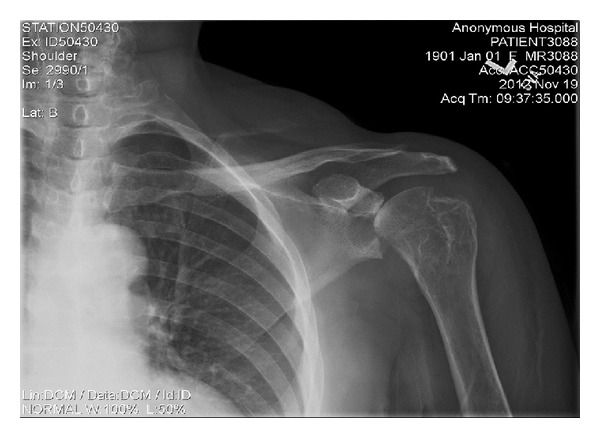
X-ray of the shoulder displaying multiple erosions of the acetabulum, humeral head, and acromioclavicular articulation.
